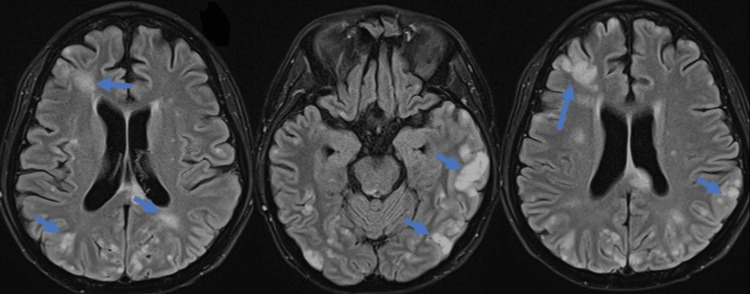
MRI brain demonstrating multiple small foci of brain infarcts in all the territory of the major vasculature, particularly in the cortical and peripheral distribution (blue arrows) MRI: magnetic resonance imaging
